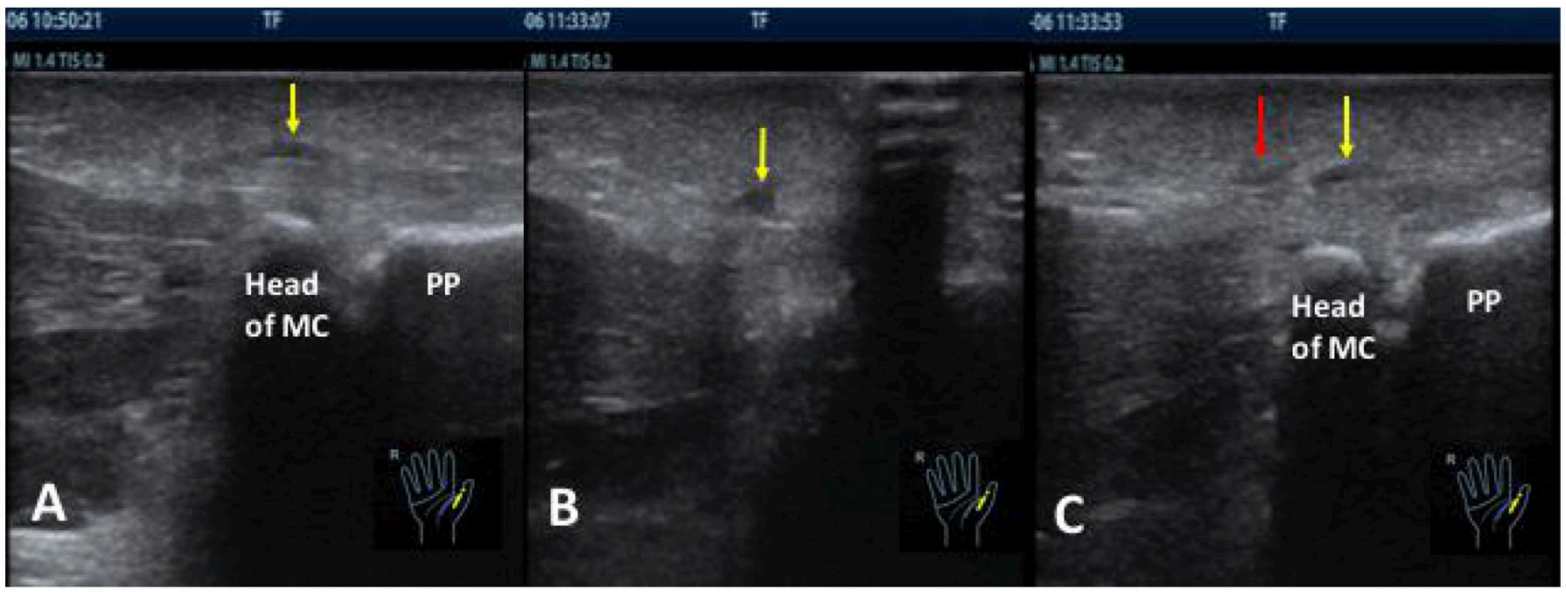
The ultrasound images before (A) and after (B,C) release in the blind group. (A) The yellow arrow showed the thickness of A1 pulley in the right thumb before release. (B) The yellow arrow showed the fluid of the surrounding tissue immediately after release. (C) The red arrow was the wrong cutting direction after piercing into the skin from the marked entry point. The yellow arrow was the thickening location of A1 pulley. They were not at the same point. PP, proximal phalange, MC, metacarpal bone.
